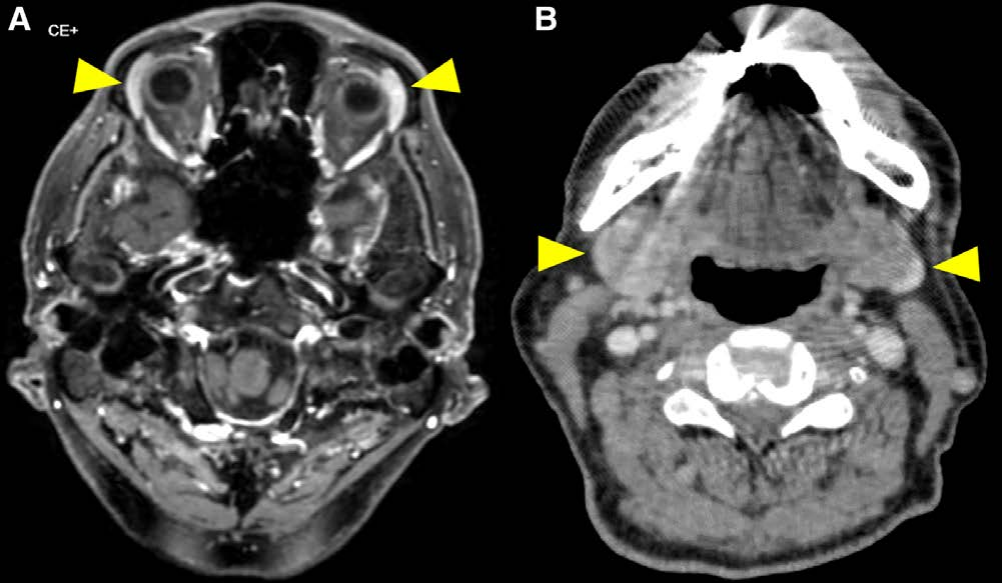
(A) Head MRI revealing swollen lacrimal glands (arrowhead). (B) Neck CT revealing bilaterally enlarged submandibular glands (arrowhead). CT = computed tomography, MRI = magnetic resonance imaging.
